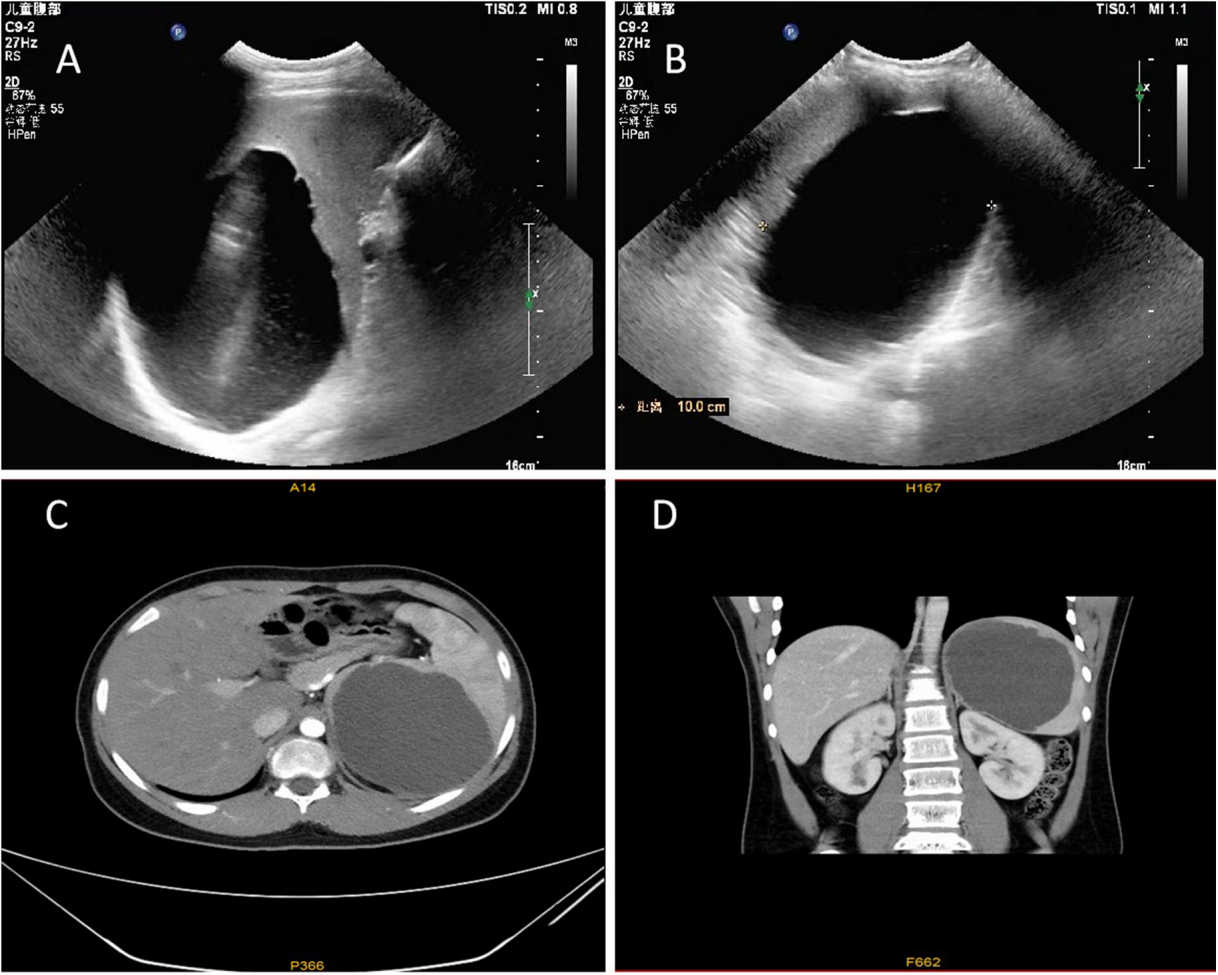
Ultrasound and CT imaging findings of splenic cyst. (A) Abdominal ultrasound findings showed a cystic echogenic area measuring approximately 11.4 cm × 11.0 cm × 10.0 cm explored at the upper pole of the spleen, with clear borders and poor internal translucency (Transverse section of the spleen). (B) Abdominal ultrasound showed the longitudinal section of the spleen. (C) Abdominal CT results showed an enlarged spleen with a cystic hypodense shadow measuring approximately 10.8 cm × 10.0 cm × 9.1 cm, with clear borders, and no obvious abnormal enhancement on enhancement scan. This figure shows a cross section of the spleen. (D) CT showed the coronal surface of the spleen.
